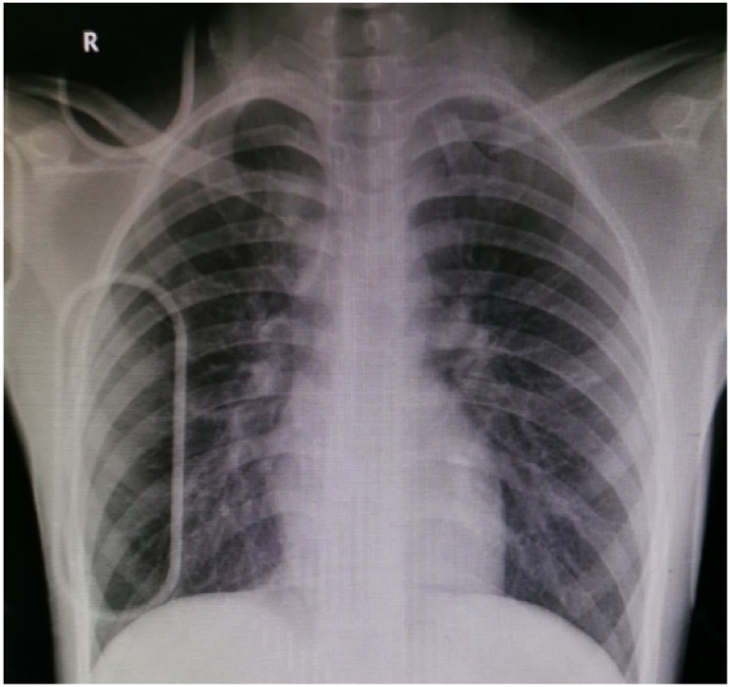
Initial anteroposterior chest radiograph demonstrating medial clavicle fracture with associated sternoclavicular joint disruption on the left side.
